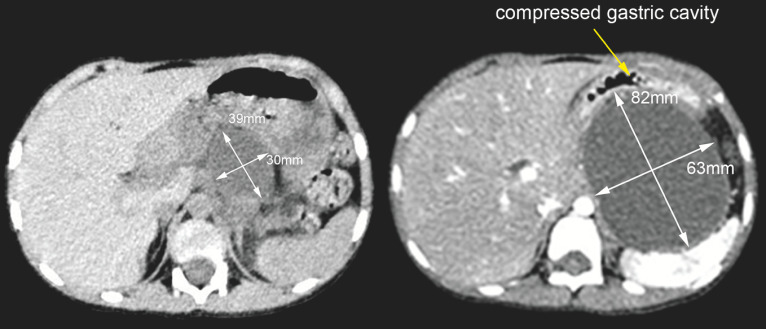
Computed tomography (CT) showed an enlarged pancreatic pseudocyst at 6-month follow-up after stent drainage of the pancreatic duct in a 4-year-old child.
